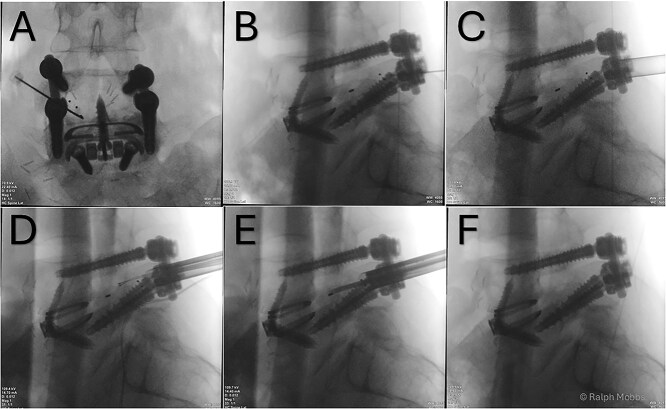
(A) AP X-ray. Initial needle localization over the TLIF cage. (B) Lateral X-ray with needle positioned on posterior aspect of TLIF cage. (C) Working channel positioned immediately posterior to cage. (D) RF diathermy to dissect around cage (see Video 1). (E) Dissection around cage using multiple instruments. (F) Post removal of cage, with no TLIF device evident.
